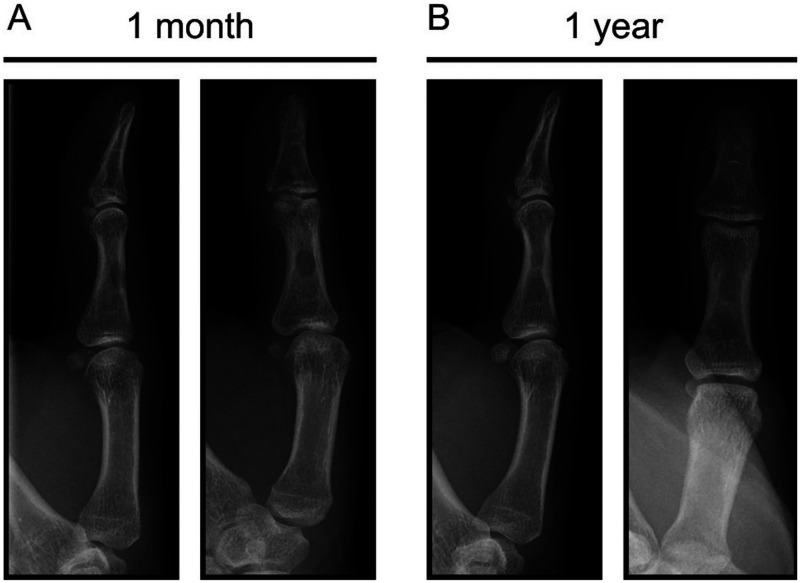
X-ray of the left thumb at 1 month (A) and 1 year (B) post-surgery.
